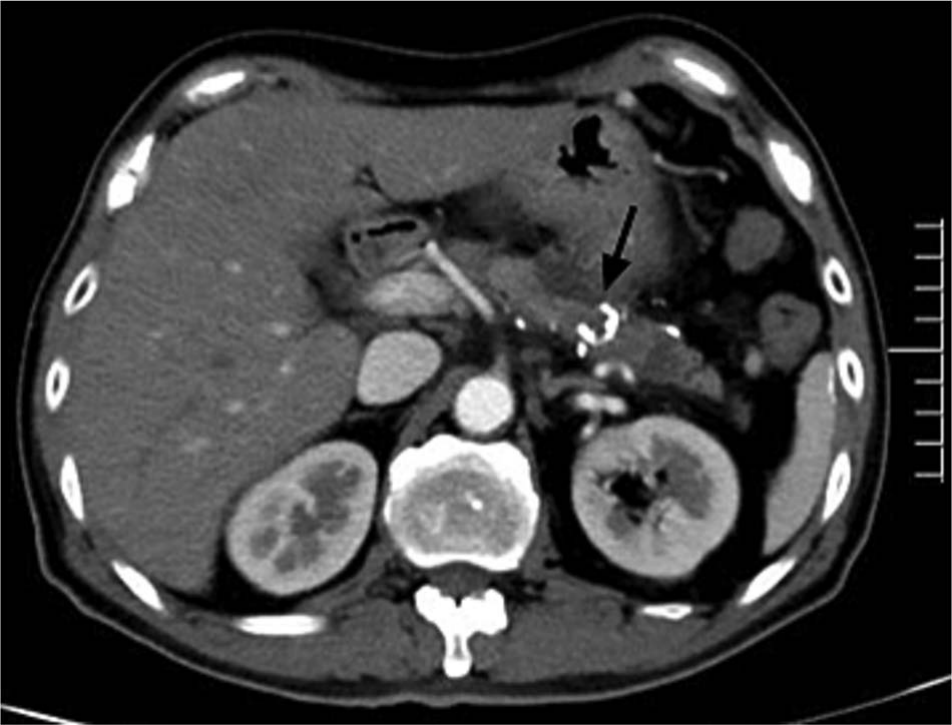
Postembolic enhanced CT after 7 months showed disappearance of PAVM with high-density spots (NBCA) in the pancreas (black arrow).
